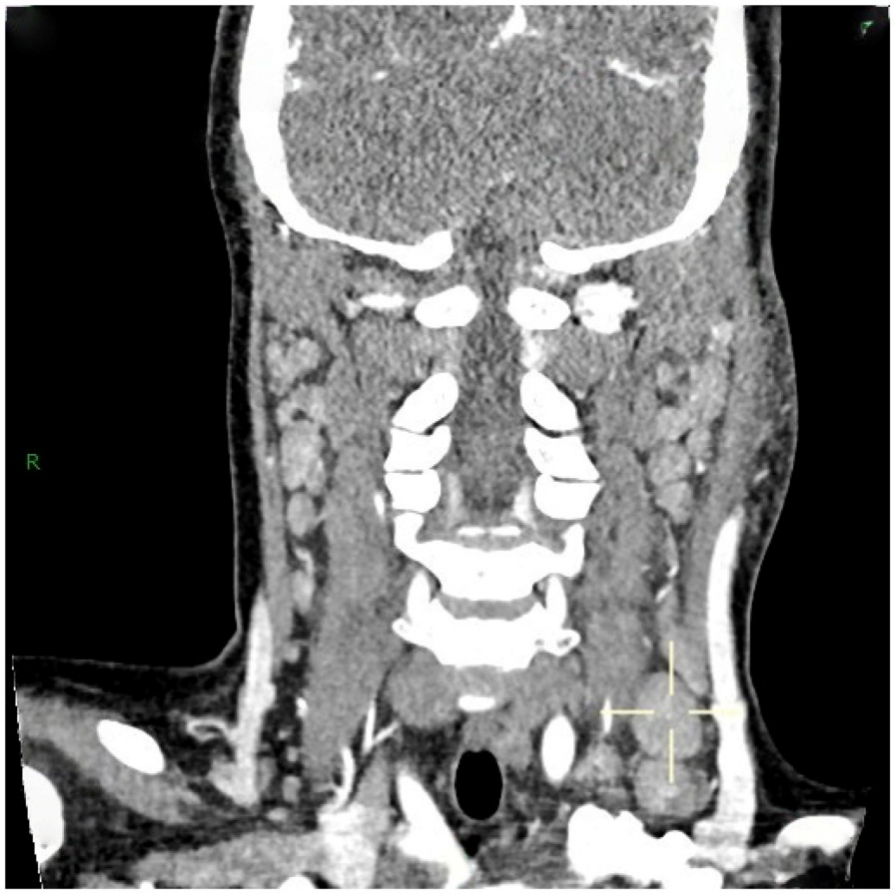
CT image of HNL lymph node.
